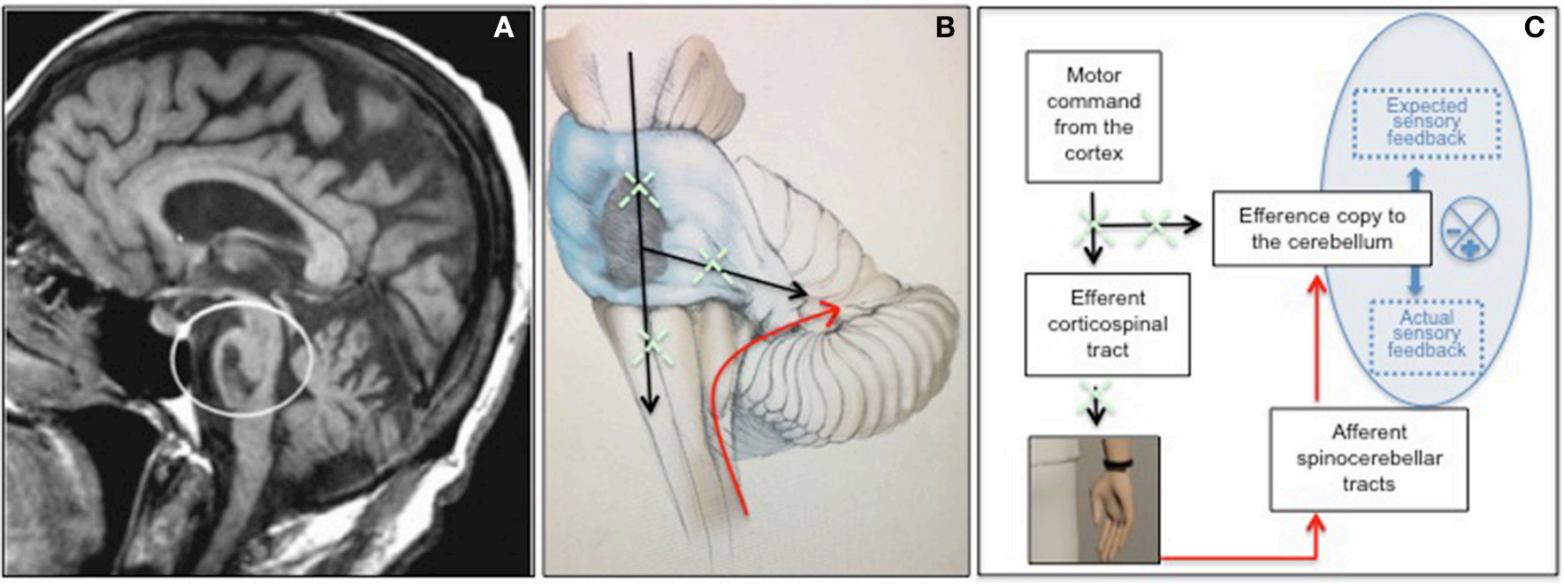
Ventral brainstem lesion in the locked-in syndrome as shown by MRI (A); graphical representation of interrupted efferent corticospinal and corticocerebellar tracts (in black) and preserved afferent spinocerebellar tracts (in red) in LIS patients (B); hypothesized mechanism by which Sonoception technology may contribute to reduce the mismatch between the efferent (defective) and the afferent (healthy) pathways in LIS and to restore properly working forward programs (C).
